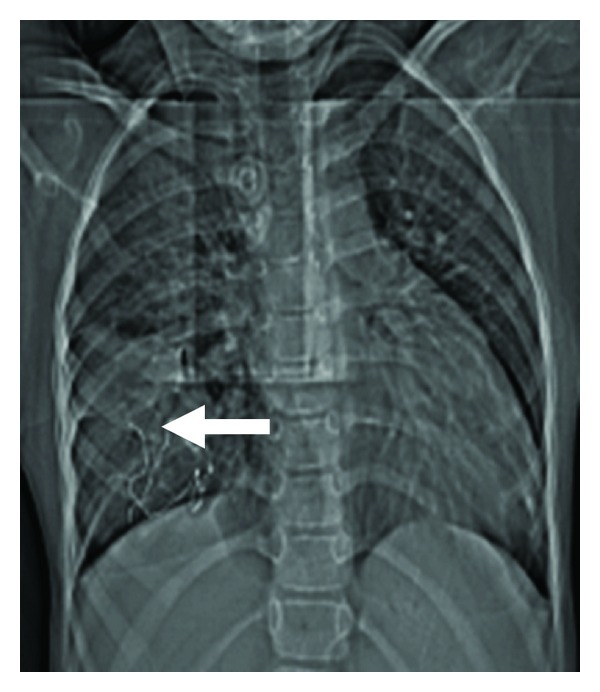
CT scanogram, showing a rounded inhomogeneous radio-opacity in the right lower hemithorax. The center of the lesion is having thin, comparatively dense lines, representing the radio-opaque markers in the retained surgical sponge (Arrow).
